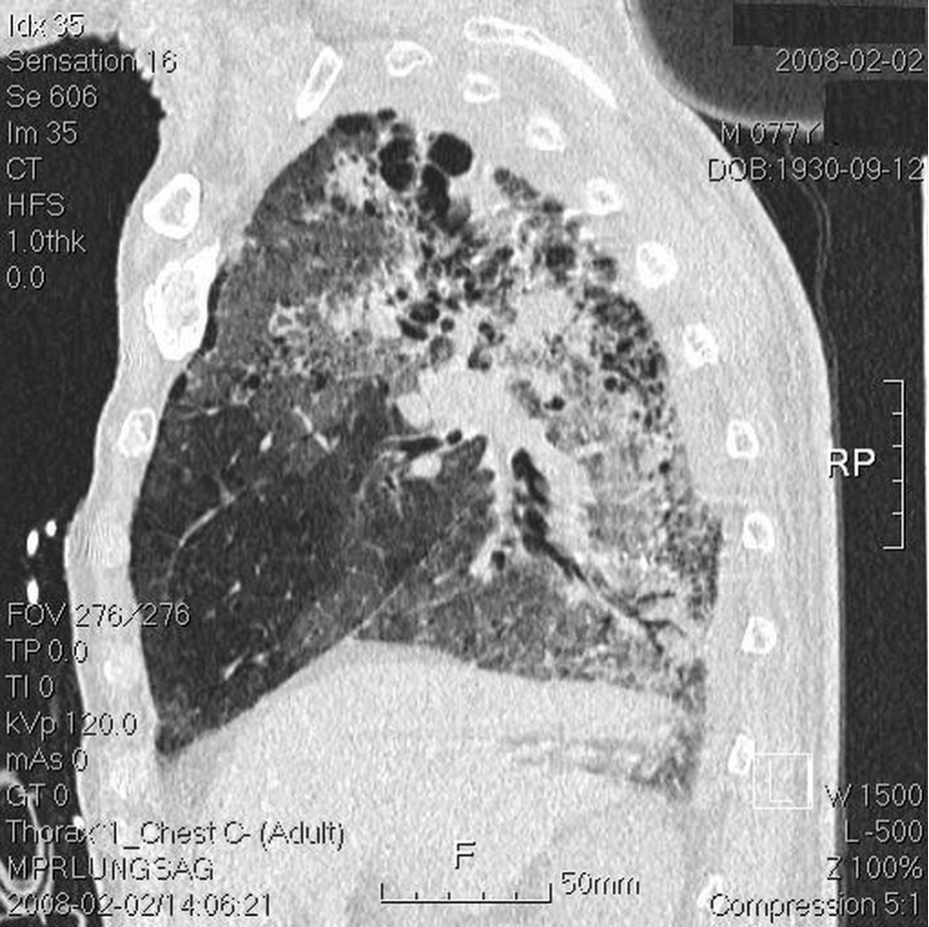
Sagittal view of the whole lung field. Chest computed tomography (CT) done post intubation shows ground-glass opacities, blebs confined to the right upper lung apex, and diffuse ground-glass attenuation, blebs in the marginal areas, airspace consolidation, and fibrosis in the bilateral upper and lower lung fields.
